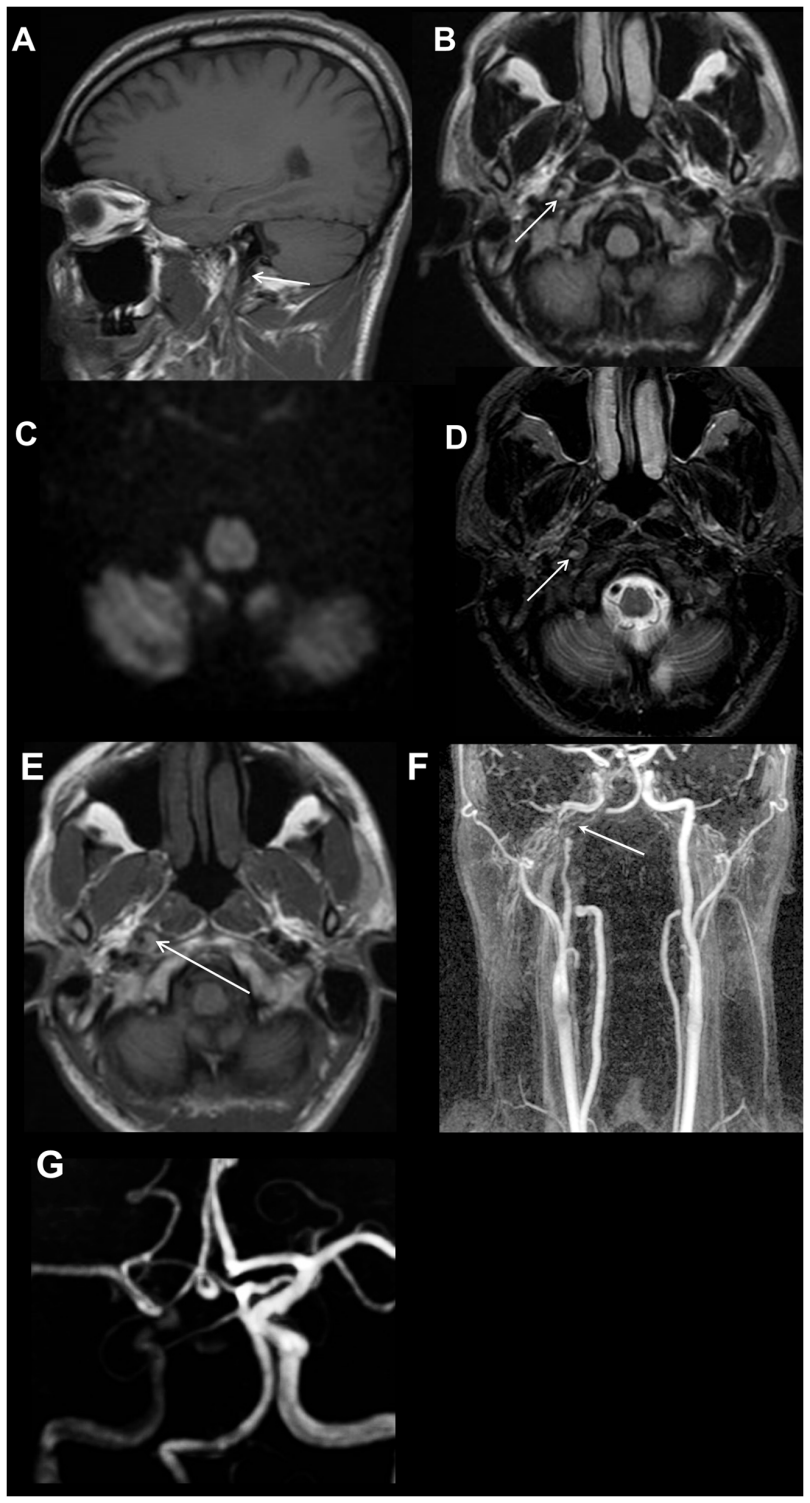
Internal carotid artery dissection in a 41 year old male with headache. The dissection was not identified on the limited 3 sequence review. Sagittal T1-weighted image (A) shows narrowing of the distal cervical portion of the right internal carotid artery with T1 hyperintense intramural hematoma (white arrow). Axial FLAIR image (B) demonstrates loss of the normal right internal carotid artery flow void (white arrow), which is more conspicuous on the axial T2-weighted image (D). Axial diffusion weighted trace image (C) shows no restricted diffusion in the region of the right internal carotid artery. Axial pre-contrast T1-weighted image (E) demonstrates an eccentric T1 hyperintense intramural hematoma (white arrow) in the distal cervical right internal carotid artery. MR angiography maximum intensity projection images reveal irregular critical narrowing (white arrow) of the distal cervical right ICA (F) and asymmetrical flow-related signal in the right intracranial ICA (G).
